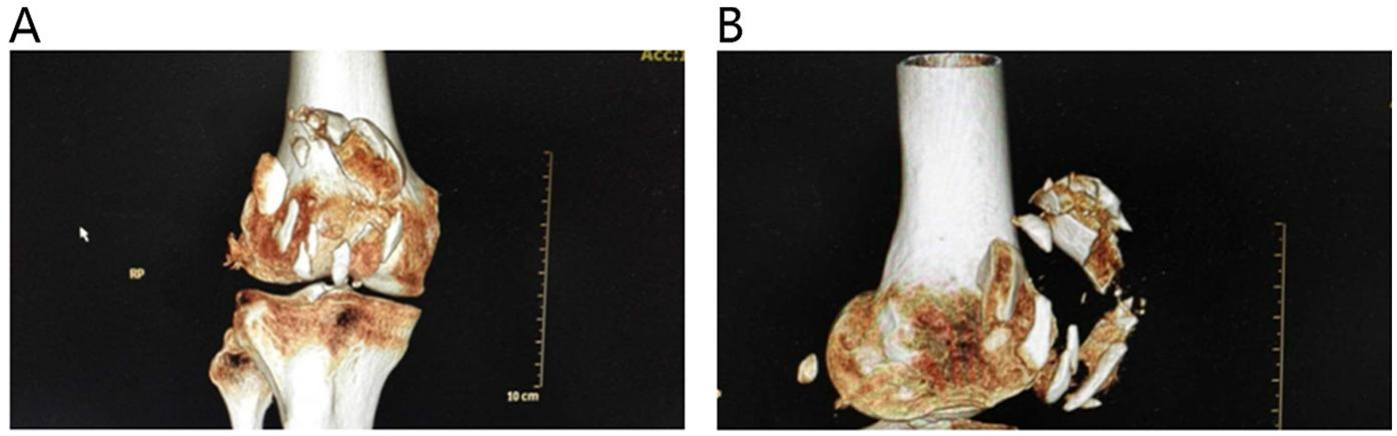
Preoperative three-dimensional reconstructed CT images of patellar fractures.
